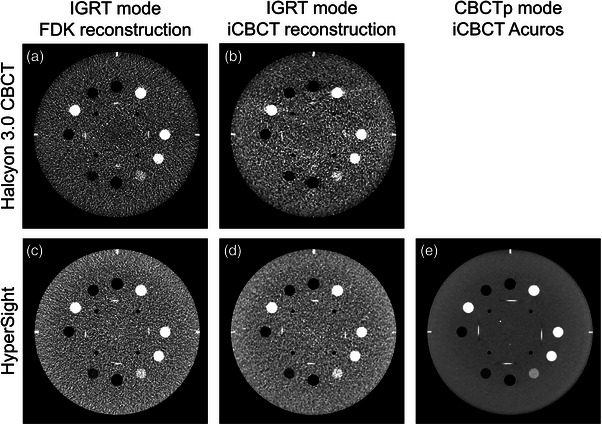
A representative CBCT image for each system, reconstruction algorithm, and mode. (a) Halcyon 3.0 CBCT IGRT mode with FDK reconstruction. (b) Halcyon 3.0 CBCT IGRT mode with iCBCT reconstruction. (c) HyperSight IGRT mode with FDK reconstruction. (d) HyperSight IGRT mode with iCBCT reconstruction. (e) HyperSight CBCTp mode with iCBCT Acuros reconstruction. CBCT, cone‐beam computational tomography; CBCTp, CBCT‐planning mode; FDK, Feldkamp–Davis–Kress; IGRT, image‐guided radiation therapy.
